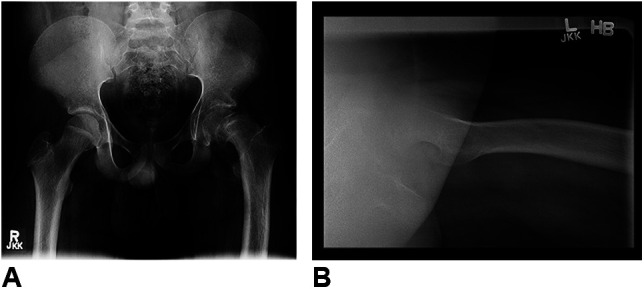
Initial radiographic investigations demonstrating a unilateral, left-sided slipped capital femoral epiphysis.
